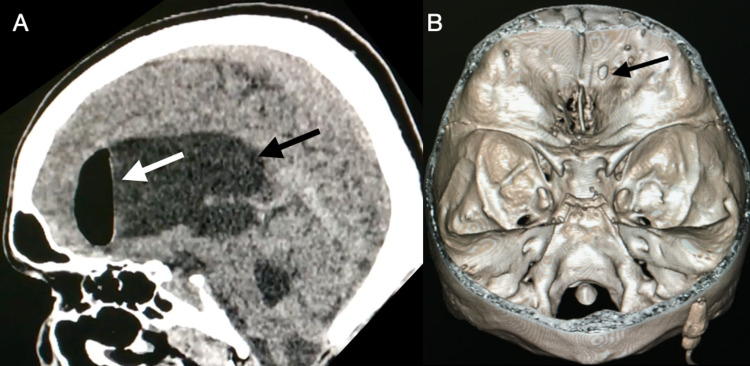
Computed tomography. (A) CT scan of skull sagittal showing intraventricular pneumocephalus (white arrow) and ventricular dilatation (black arrow); (B) three-dimensional computed tomography reconstruction showing left frontobasal bone defect (black arrow). CT: computed tomography.
